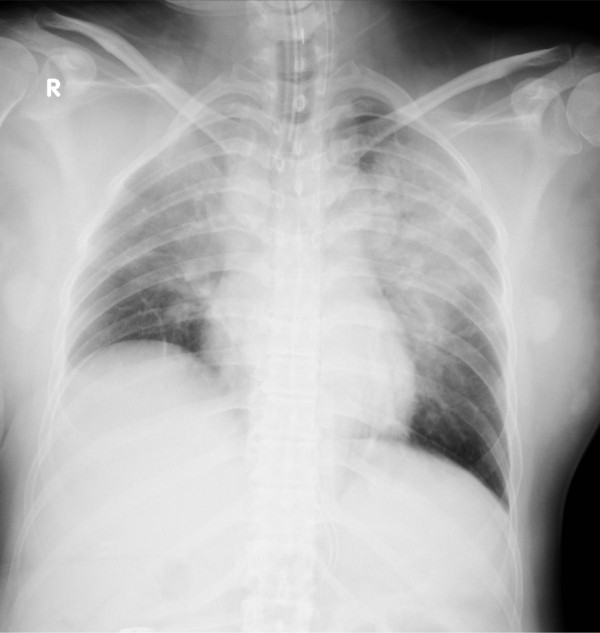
A chest X-ray of the mother reveals consolidation at bilateral upper lung field.
